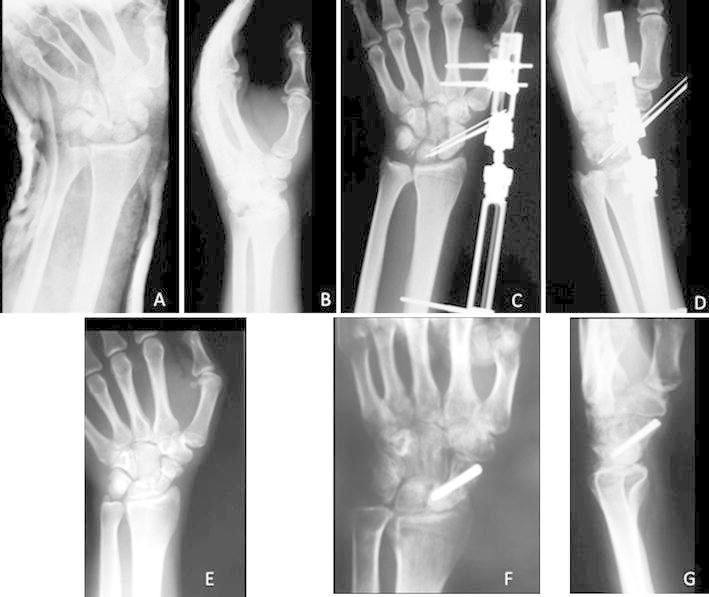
Posteroanterior (a) and lateral (b) views of the left wrist of a 35-years-old male with a trans-scaphoid perilunate fracture dislocation. Posteroanterior (c) and lateral (d) radiographs after open reduction through a dorsal approach, application of a wrist external fixator and K-wires. The scaphoid was fixed with K-wires. e Three months postoperatively, a scaphoid nonunion was radiographically evident. A cannulated screw was used for the scaphoid nonunion. Posteroanterior (f) and lateral (g) radiographs of the wrist 10 months postoperatively showed the development of post-traumatic
